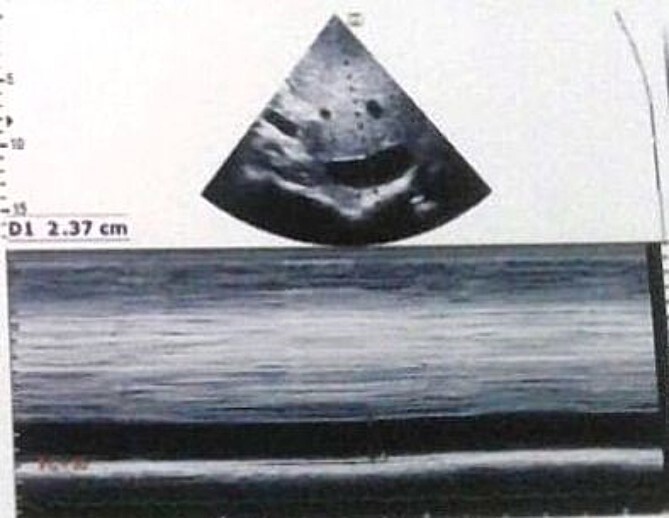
Transthoracic echocardiography showed inferior vena cava dilation.
